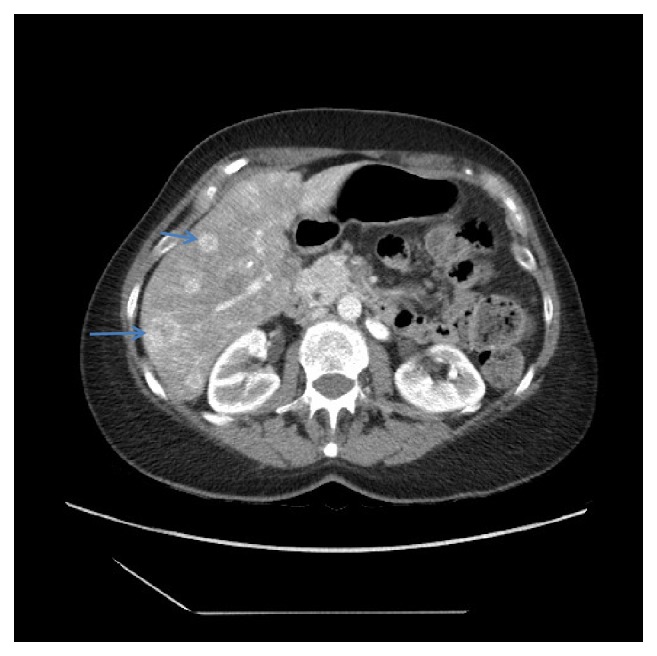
Computed tomography image showing multifocal hepatic lesions with peripheral enhancement and central hypoattenuation. Blue arrows point to calcifications.
